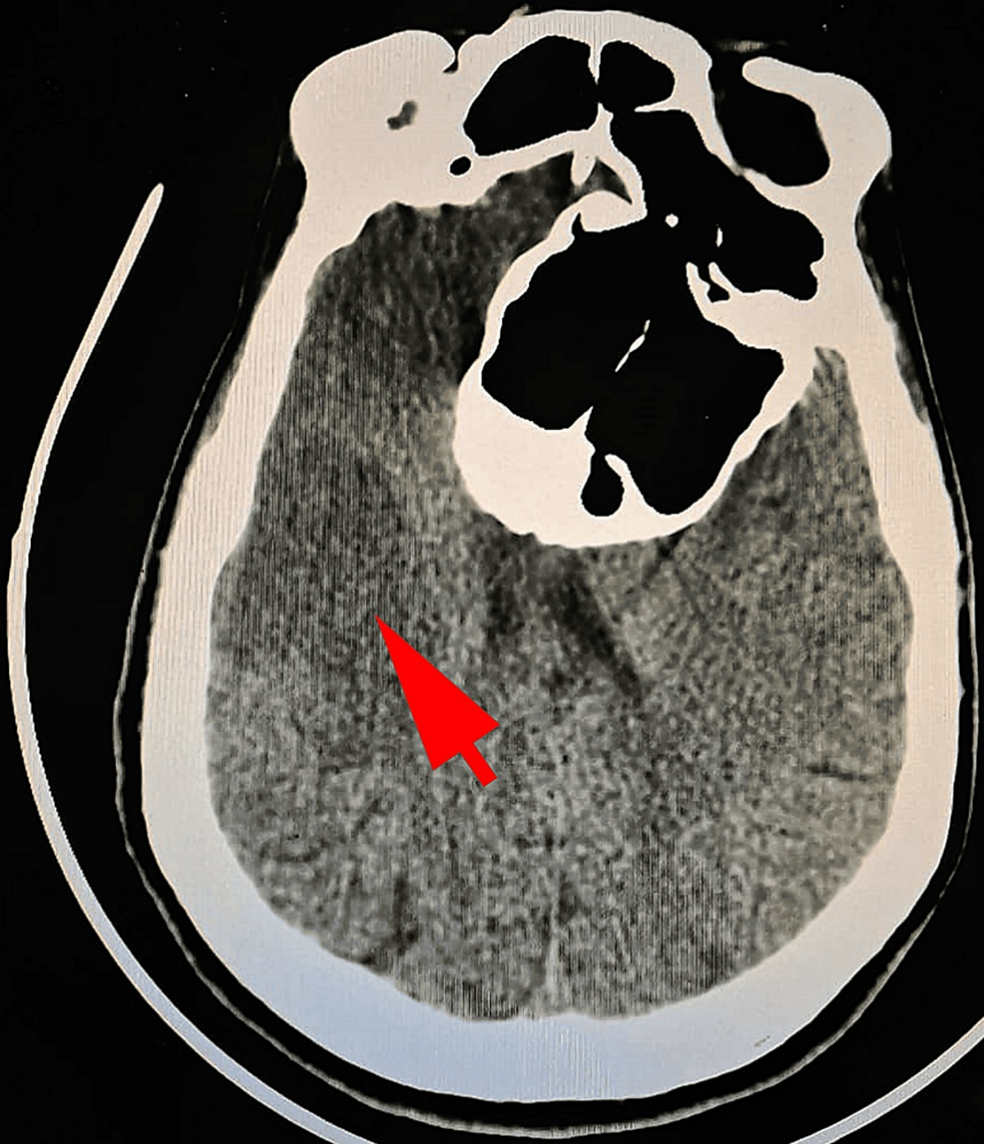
The computed tomography (CT) scan revealed spontaneous hypodensity (arrow) on right cerebral medial artery with loss of grey-white matter differentiation and gyral effacement
